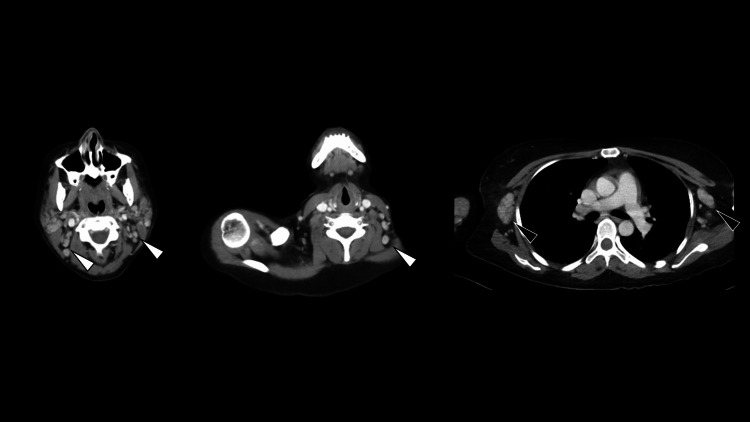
Contrast-enhanced computed tomography of the whole body at presentation Computed tomography demonstrates generalized lymphadenopathy in cervical (white arrowheads) and axillary regions (black arrowheads).
